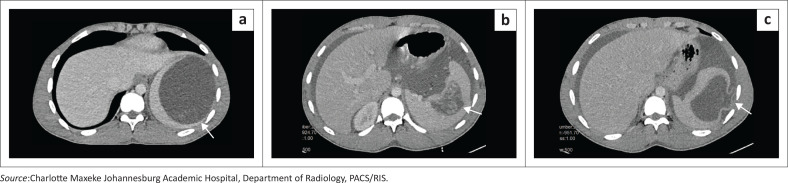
Ruptured splenic hydatid cyst in a 16-year-old patient presenting with acute abdominal pain. (a) Axial contrast-enhanced CT demonstrates a unilocular cystic splenic lesion with hyperdense ill-defined dependent internal contents (arrow). (b, c) Additional axial CT images demonstrate detached internal membranes within the cyst, characteristic of hydatid disease (arrow in b). Focal thinning and irregularity of the adjacent splenic parenchyma suggests the site of rupture (arrow in c), with associated large-volume ascites and no peritoneal deposits or additional sites of disease.
